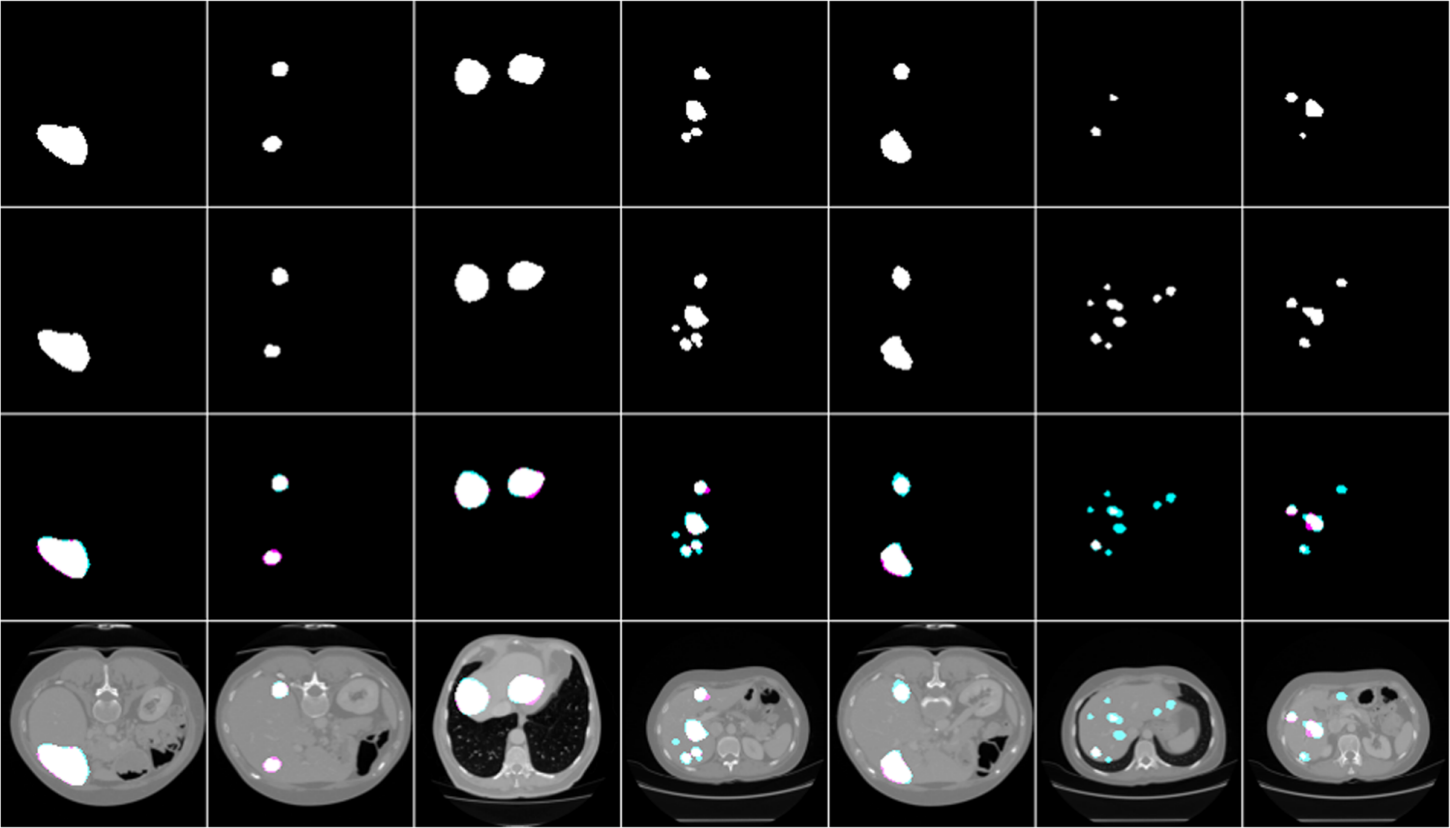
Result of tumor segmentation from abdominal CT images with the respective masks and overlap images
